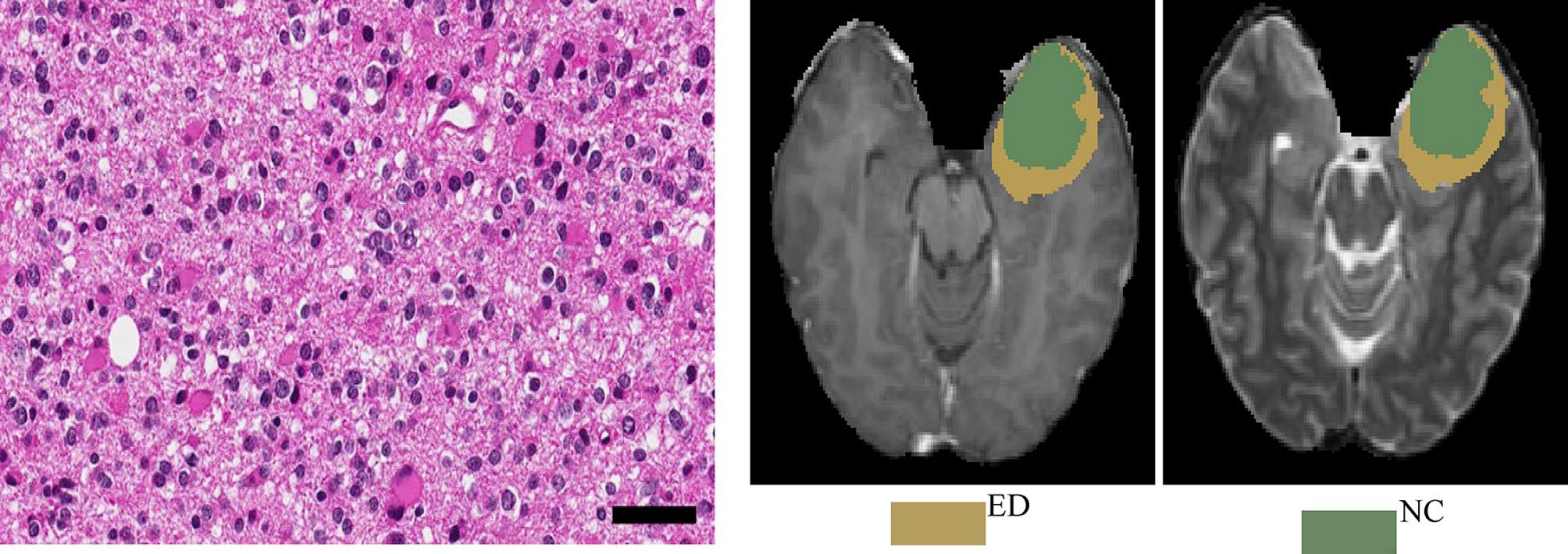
One of the cases that was overdiagnosed by the WSI-based approach but successfully corrected by the MRI-based approach after the proposed label agreement scheme. Left: Pathological observation. Right: The brain tumor segmentation on MRI.
